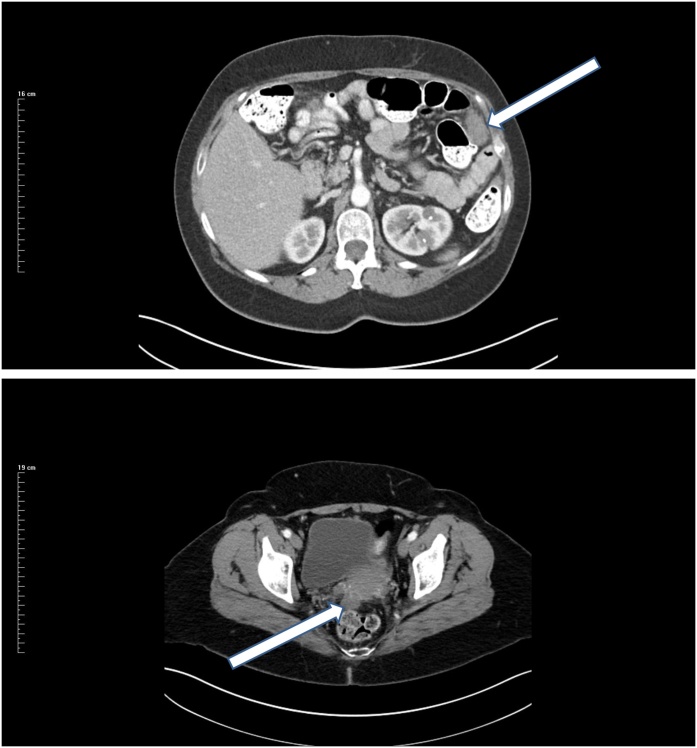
(Top) CT slice through the mid-abdomen at one year follow-up shows tumor nodules thought to be located in the greater omentum. (Bottom) CT slice through the pelvis shows a 4 cm mass in the rectouterine (cul de sac) space.
